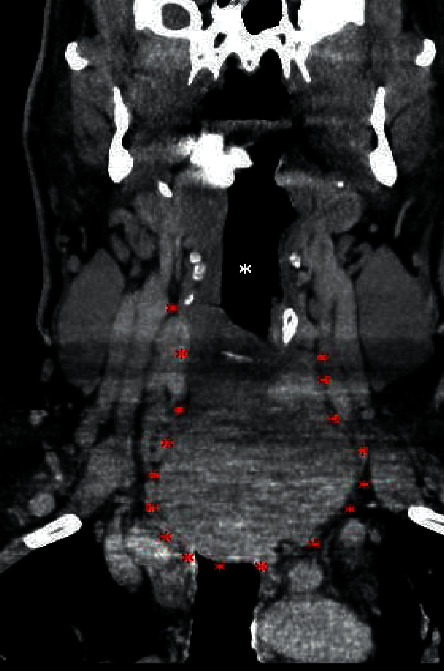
Enhanced coronal computed tomography image demonstrating a large thyroid mass (red asterisk) with invasion of the superior mediastinum. The airway (white asterisk) is displaced outside the field of view of this image.
